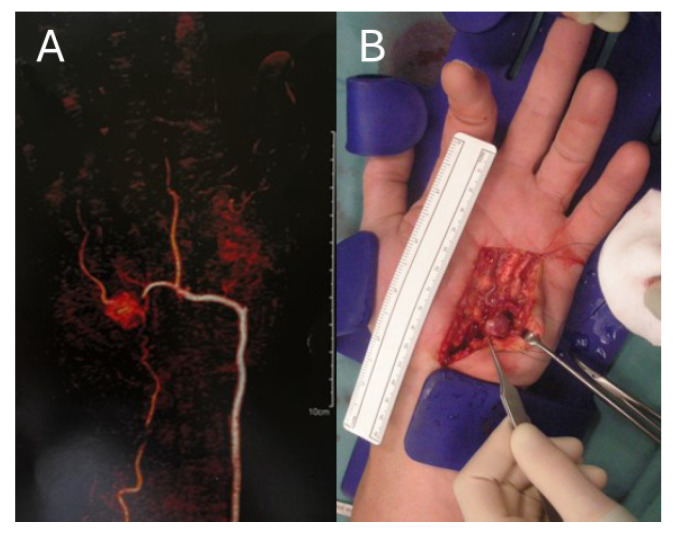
Left hand of a 62-year-old male with an arterio-venous malformation in the hypothenar region; (A) CT-angiography with a contrasting agent which shows an arterio-venous malformation of the left hypothenar; (B) arterio-venous malformation during surgical resection.
